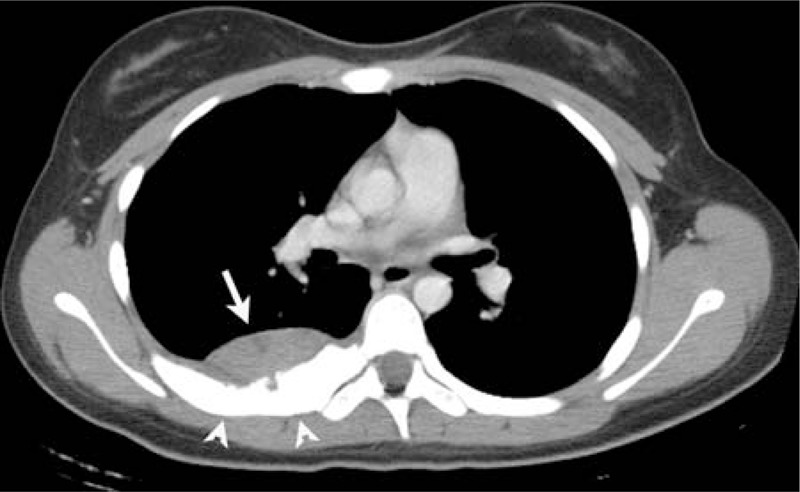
A 19-year-old woman presented with a history of right chest pain for about 1 month. Enhanced CT scan shows that the tumor had mild heterogeneous enhancement in the posterior right chest wall (arrow) and bony destruction (arrowhead).
